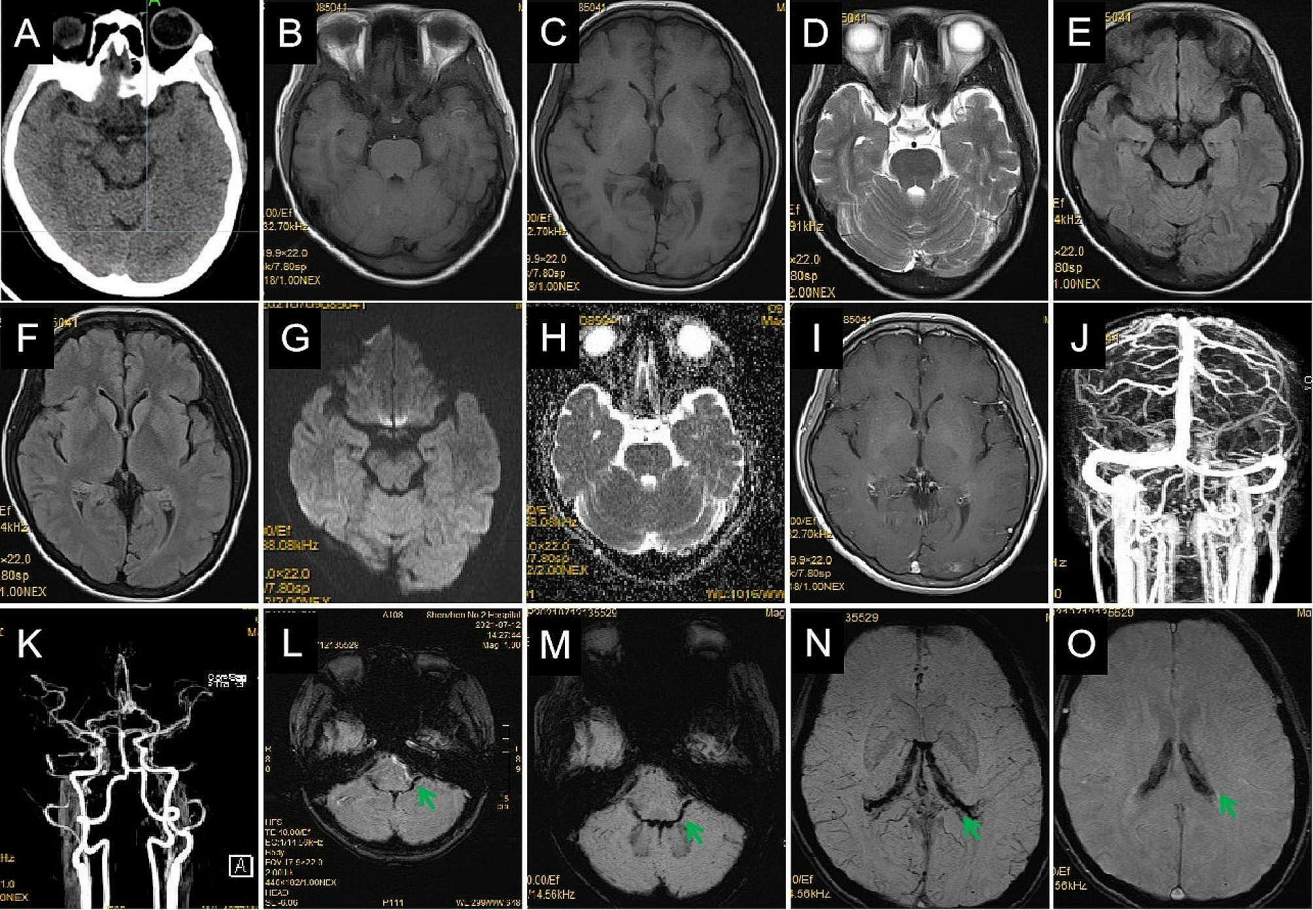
The patient’s CT and MR imaging findings. The initial brain CT (A), brain MR imaging (B and C, T1-WI; D, T2-WI; E and F, fluid-attenuated inversion recovery sequences; G, diffusion-WI; H, apparent diffusion coefficient), enhanced MR imaging (I), MR venography (J), and MR arteriography (K) show no obvious abnormalities. Susceptibility-weighted imaging (L-O) shows abnormal signals in bilateral ventricles, the fourth ventricle, and the brainstem surface. CT, computed tomography; MR, magnetic resonance; WI, weighted imaging
